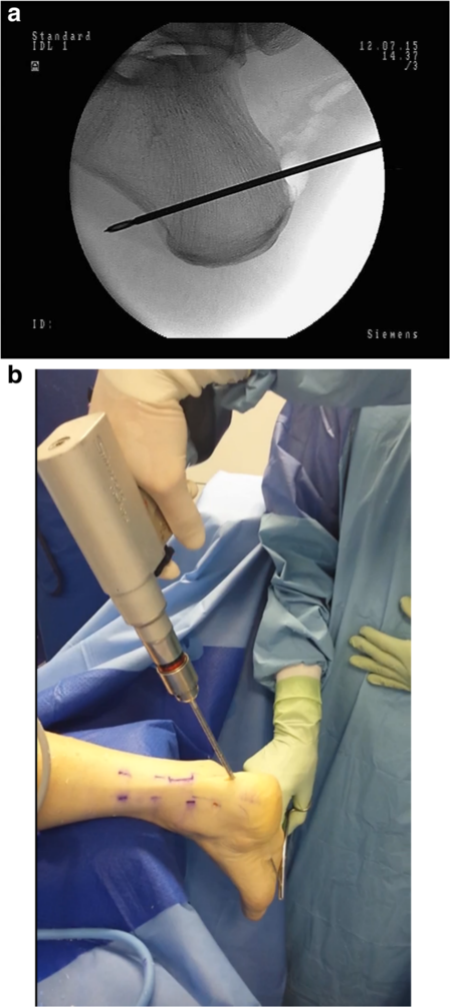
a. X-ray control of K-wire placement. b. Calcaneus drilling using skin incision No 1
